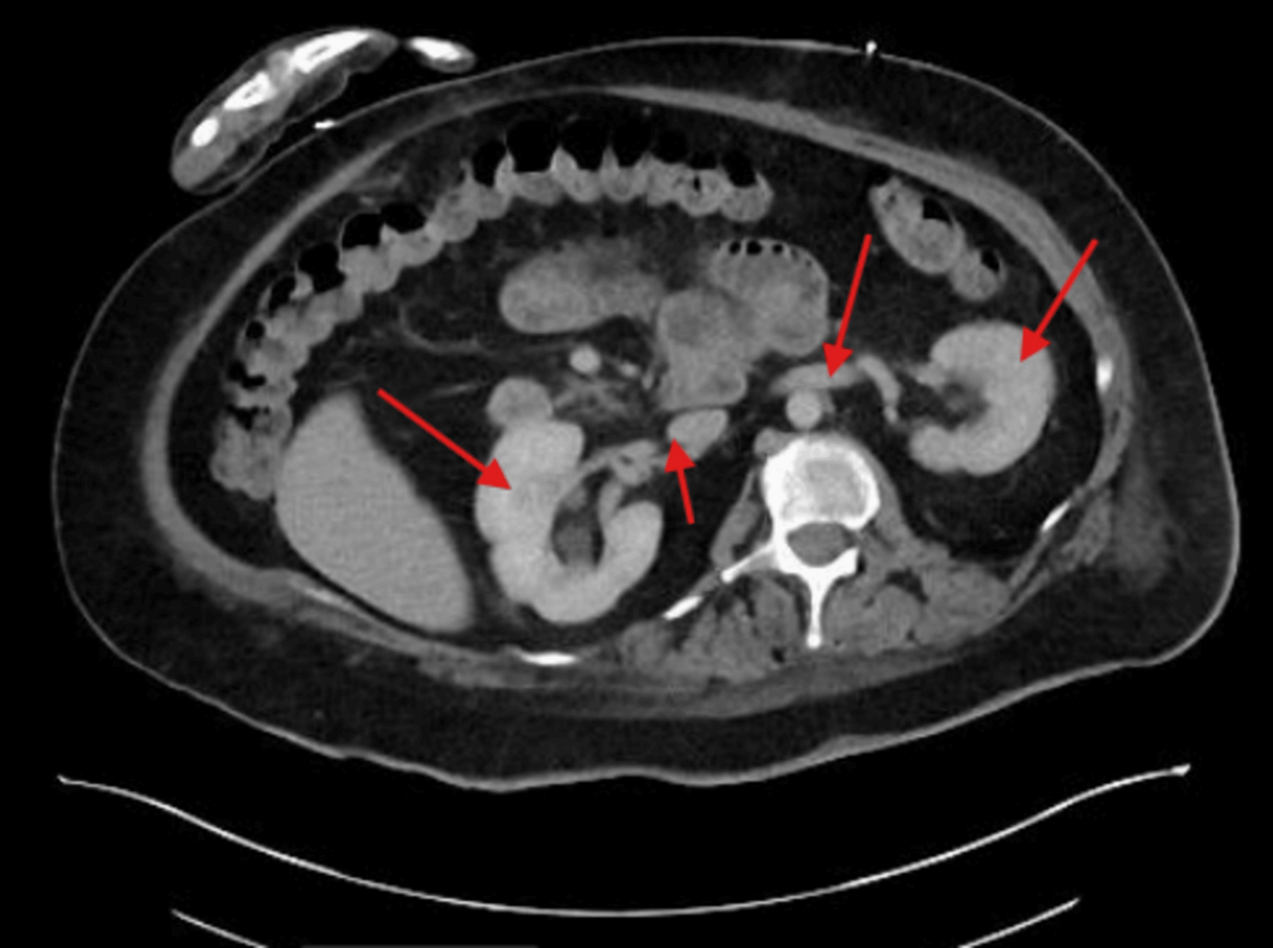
CT of abdomen and pelvis with IV contrast depicts bilateral hydronephrosis without an obstructing stone. Red arrows point out the bilateral hydronephrosis.
